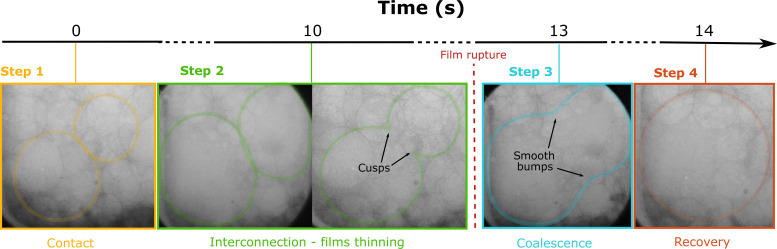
Radiographic images of Superliq_Dec runs showing coalescence steps and timescales. (1) contact between two bubbles; (2) bubble interconnection during which bubble walls thin (bubbles assume an “eight” shape with cusps on their walls); (3) bubble coalescence after the rupture of the thinned films (replacement of the cusps in the “eight” shape by smooth bumps on the walls of the new coalesced bubble); (4) bubbles assume an oval shape and lastly recover to a spherical one.
